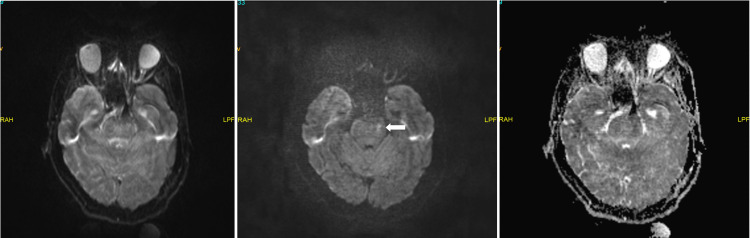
Brain magnetic resonance imaging. Diffusion sequences (b0 and b1000) and apparent diffusion coefficient (ADC) map, respectively. Note the small focus of diffusion restriction on the left cebebral peduncle (arrow).
